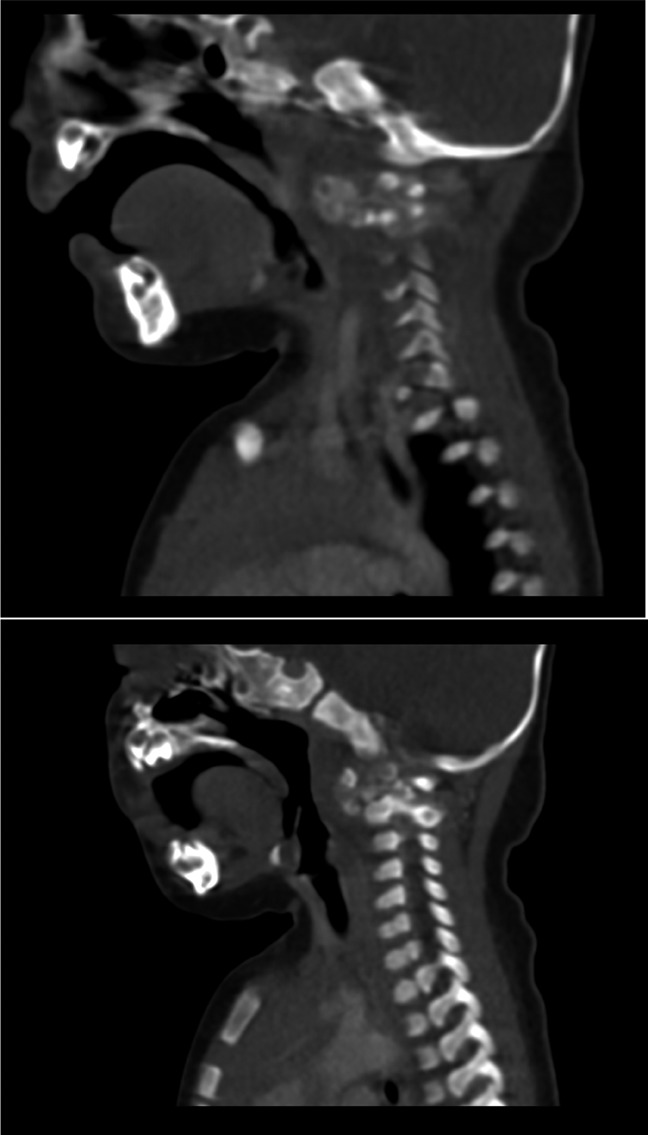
CT scan showing tumoral calcinosis. CT scan revealing the patient's tumoral calcinosis on initial presentation. Abnormal calcification and edema are seen in the retropharynx and around the C1/C2 vertebrae articulation.
